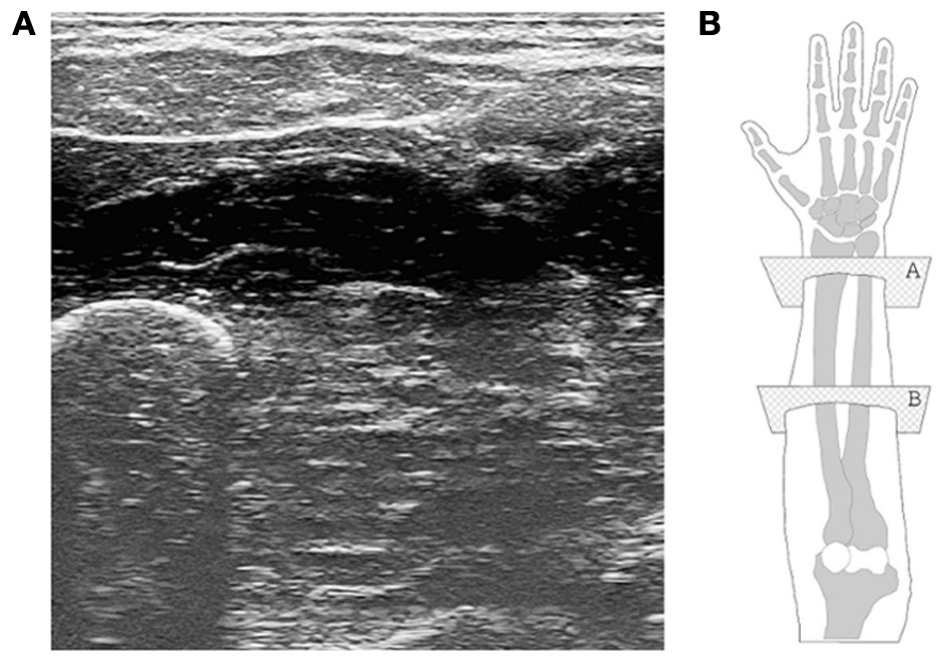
(A) A typical ultrasound image obtained during the experiment. The ulna is clearly visible in the bottom-left corner, while the flexor muscles and tendons are seen in the upper part. (B) A graphical representation of the human hand and forearm (right forearm; dorsal side up). The transducer is placed onto the *ventral* side; plane “B” corresponds to the section from which the ultrasound image was taken.
